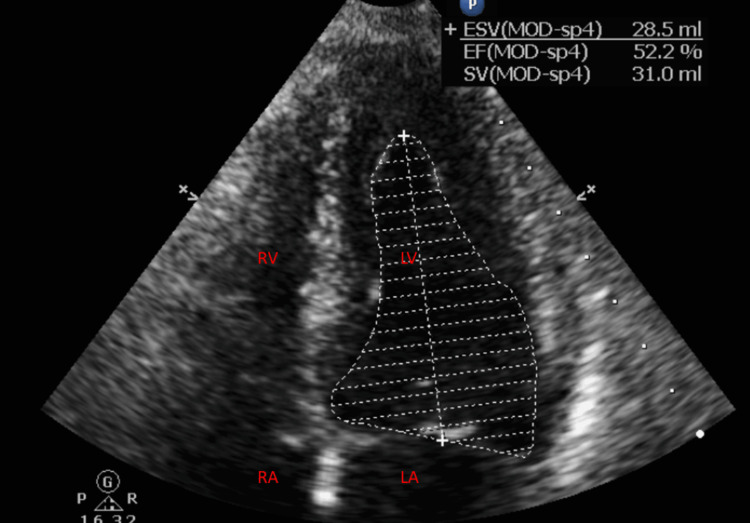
Transthoracic echocardiogram depicting the heart of the patient returning to normal size and with normal ejection fraction. LV-Left Ventricle, LA-Left Atrium, RV-Right Ventricle.
